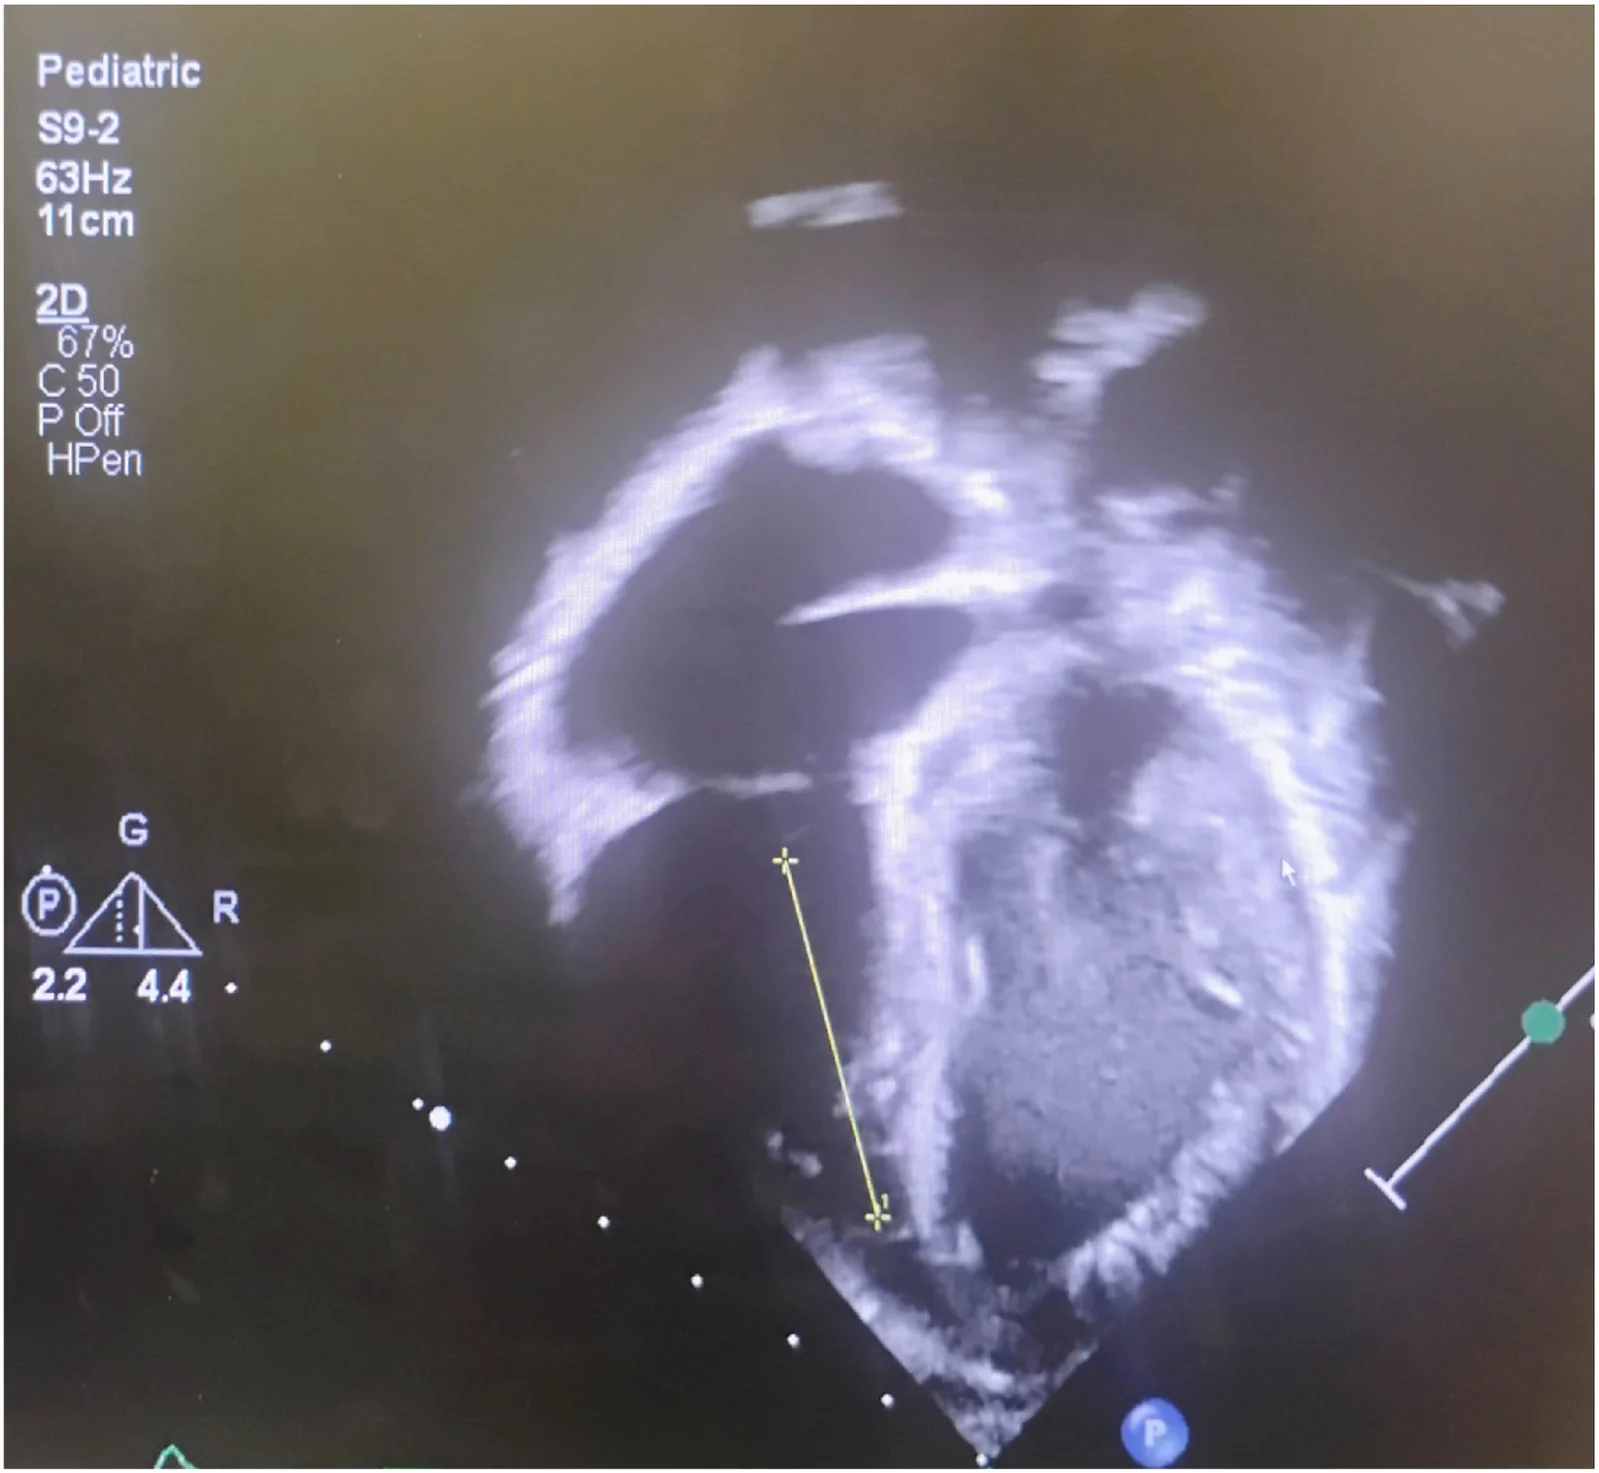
Four chamber apical view via transthoracic echocardiogram prescreening measuring right ventricle dimensions.
